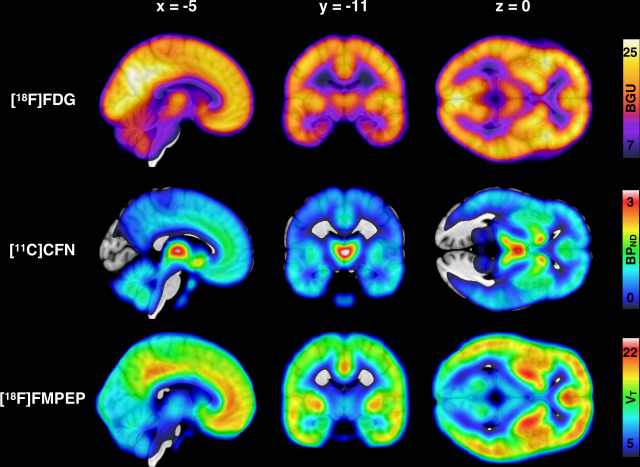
Mean distribution of brain glucose uptake, μ-opioid receptor availability and CB_1_ receptor availability in the whole study sample. Top: Mean brain glucose uptake (BGU) of the 38 [^18^F]FDG scans (19 low-risk and 19 high-risk subjects). Middle: Mean binding potential (*BP*_ND_) of the 41 [^11^C]carfentanil scans (22 low-risk and 19 high-risk subjects). Bottom: Mean volume of distribution (*V*_T_) of the 36 [^18^F]FMPEP-*d*_*2*_ scans (20 low-risk and 16 high-risk subjects).
